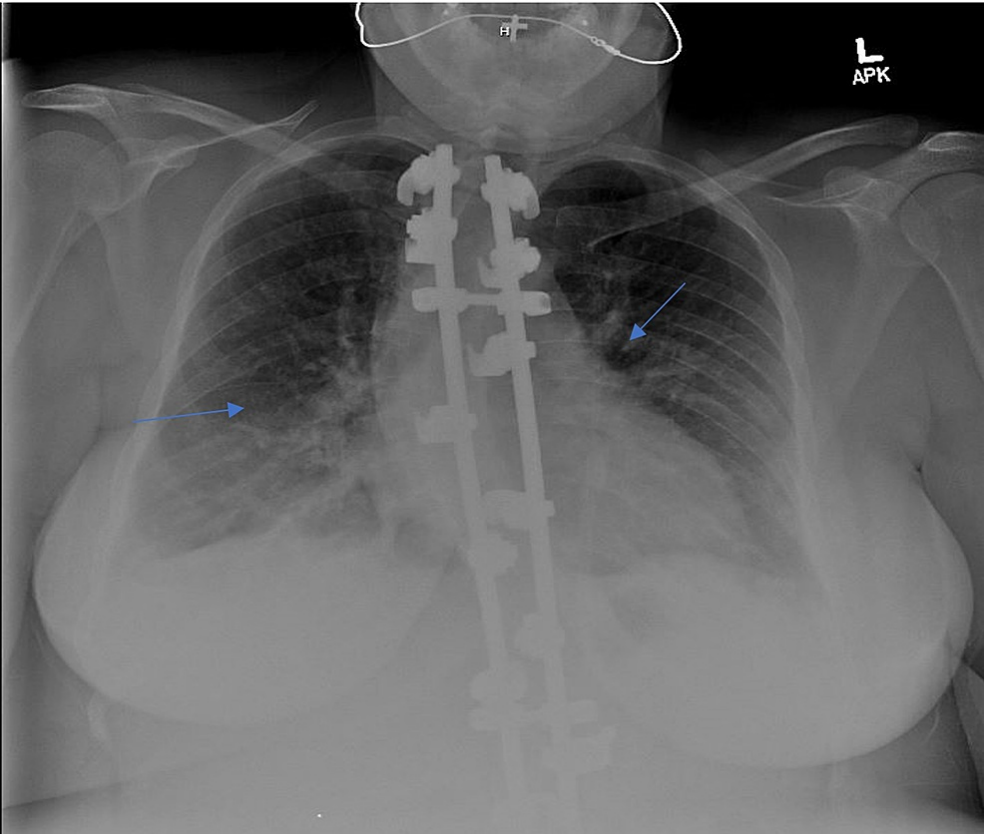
Chest X-ray showing pulmonary edema with bilateral vascular congestion with mild interstitial thickening (arrows)
